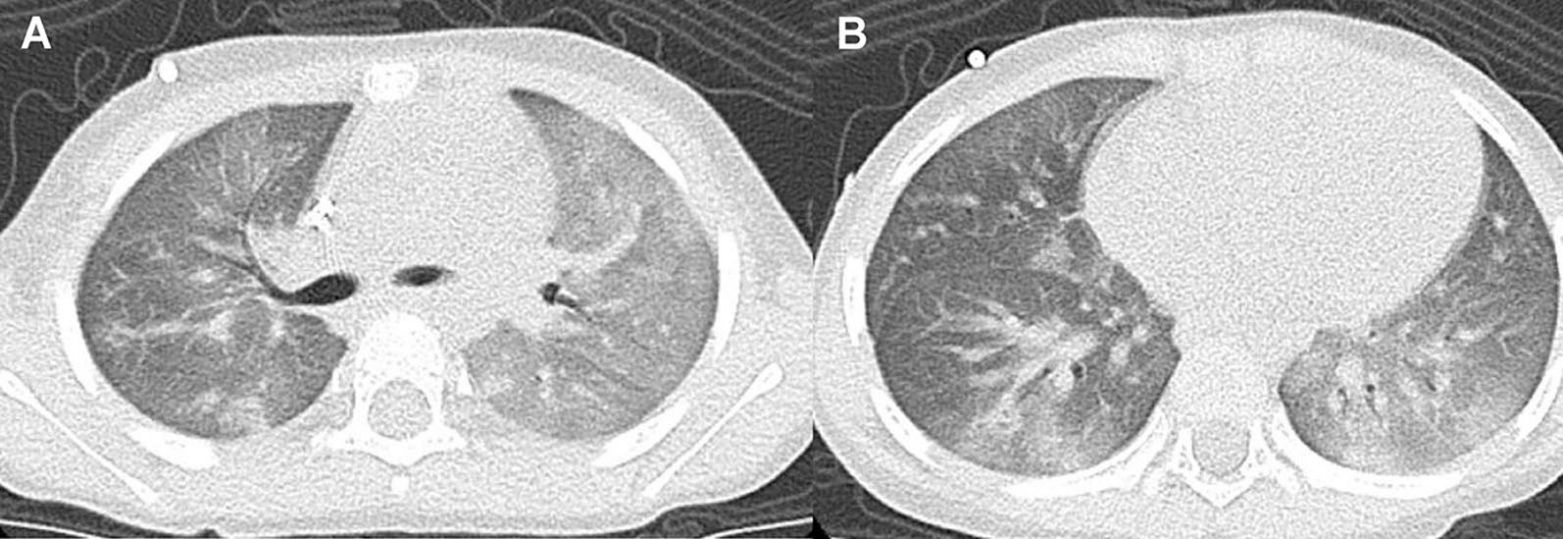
Post-transplant alveolar hemorrhage. A 3-year-old girl who developed hemoptysis after autologous peripheral blood stem cell transplantation following chemotherapy for neuroblastoma. A,B CT showed extensive ground glass shadows with “dark bronchus sign” (clearly depicted bronchial translucency due to alveolar blood filling)
